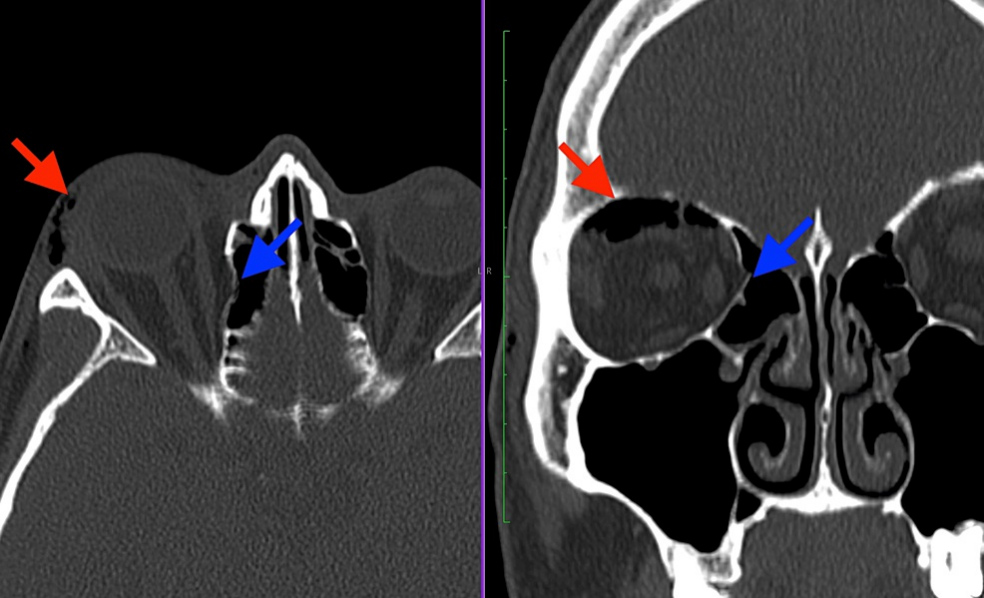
Axial and coronal view of the CT bone window showed focal indentation of the right lamina papyracea (blue arrows) and right orbito-palpebral emphysema (red arrows) similar to the tissue window but showed neither gap at the wall of lamina papyracea nor orbital tissue prolapse, which is suggestive of dehiscence as opposed to fracture.
